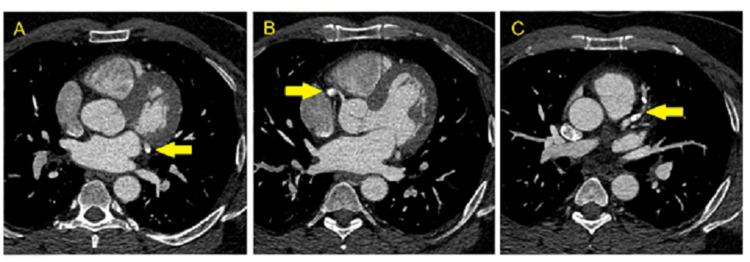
CTCA demonstrating (A) left circumflex artery with proximal plaque, (B) RCA with mid-segment focal restenosis and plaque, (C) LAD artery with ecstatic changes, mid, and distal plaques. CTCA: Computed Tomography Coronary Artery, RCA: Right Coronary Artery, LAD: Left Anterior Descending
